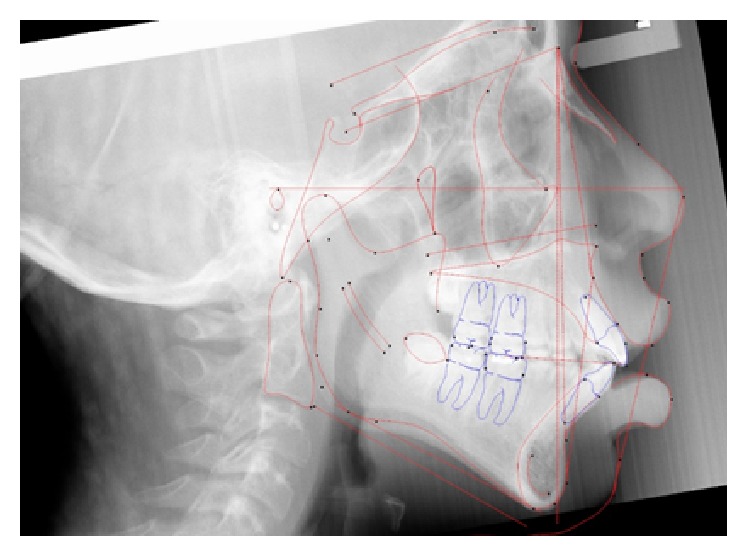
Stage 1: Orthodontic Treatment Cephalometric Radiograph.
